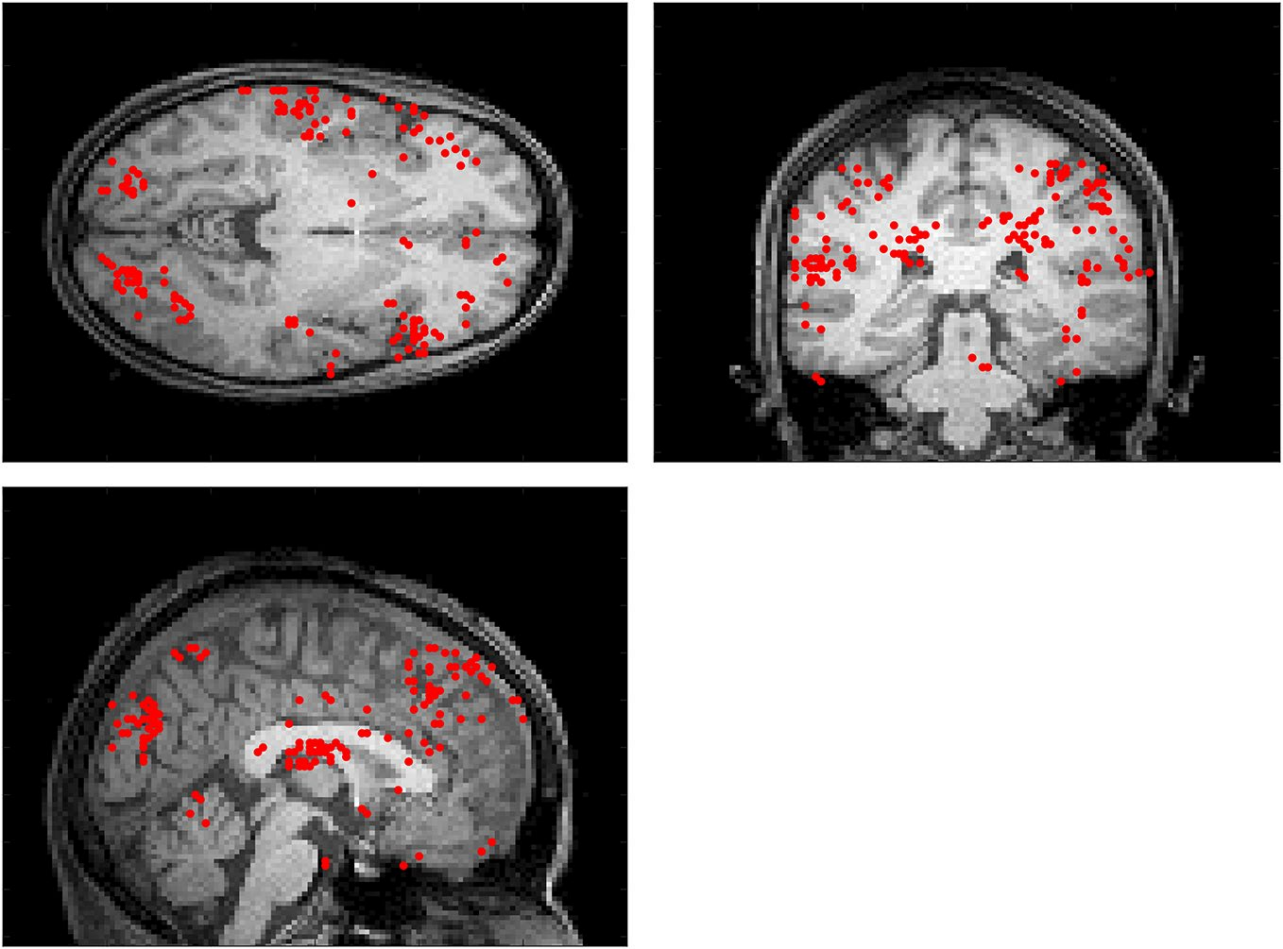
Locations of the 4 dipole locations chosen for each fold of the $$3~\times ~3$$ cross fold validation scheme for each participant and overlaid on a standard T1-weighted image projected on the axial, coronal, and sagittal planes.
